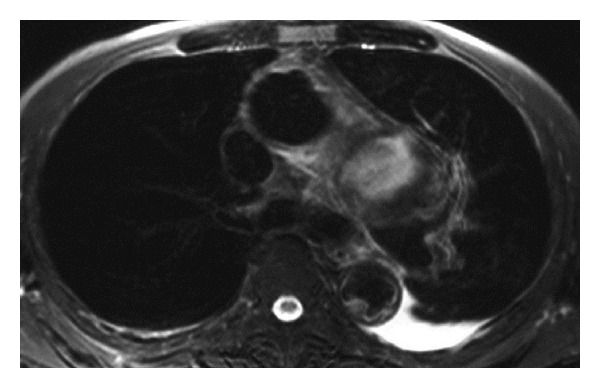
Transverse MRI (T2-weighted image) of the chest revealing a thoracic aortic saccular aneurysm protruding downward in the aortopulmonary window.
